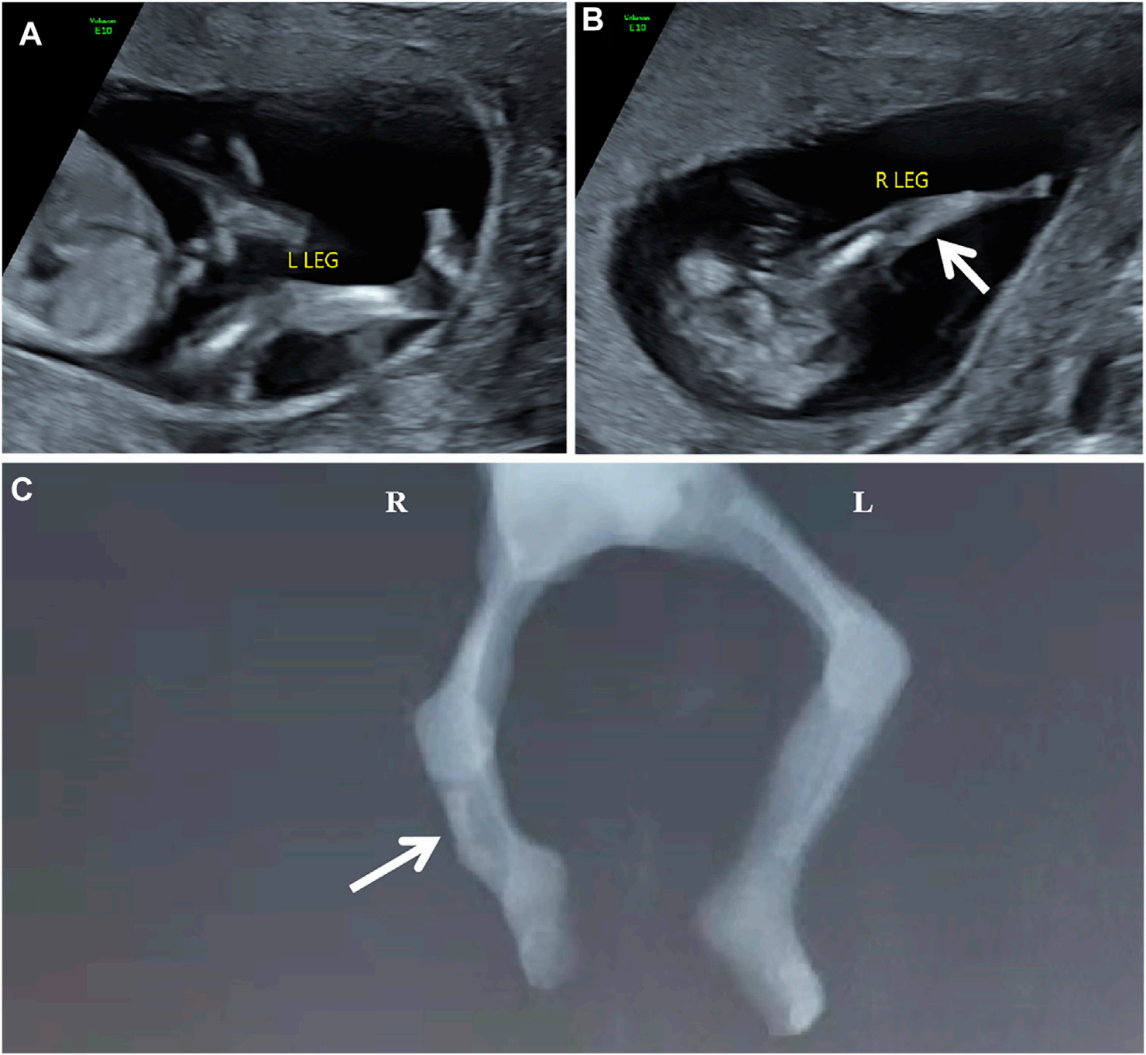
The scan images of the proband [(A,B) the ultrasonography at 13+6 weeks of gestation showed abnormal development of the right calf. (C) the X-ray of the proband showed the absence of the right fibula with the bent tibia.]
